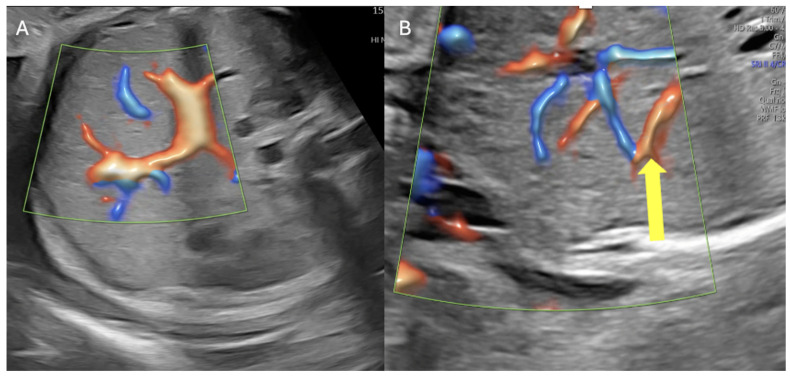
Intrauterine undernutrition: intrahepatic portosystemic shunt in fetal growth restriction. (A) Normal fetal portal venous anatomy. (B) Ultrasound image at 26 weeks’ gestation demonstrating an intrahepatic portosystemic shunt (yellow arrow) in a fetus with growth restriction, that may lead to ischemic changes to the fetal liver, who is prone to develop future complications as a result [94].
